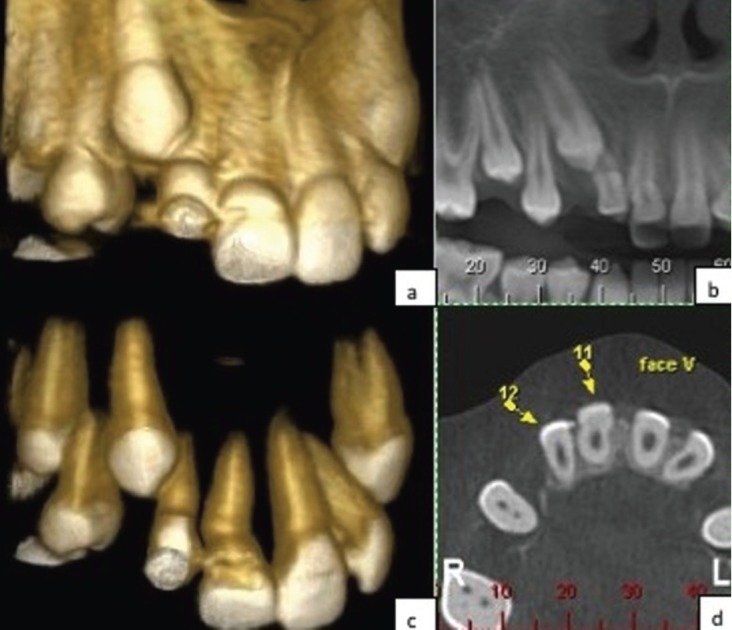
Cone beam computed tomography showing the enamel bridge between central and right lateral incisor still stayed after a first attempt of separation. (a) Vestibular view (b) Panoramic view (c) Vestibular view with removal bone tissue (d) Axial cutting.
